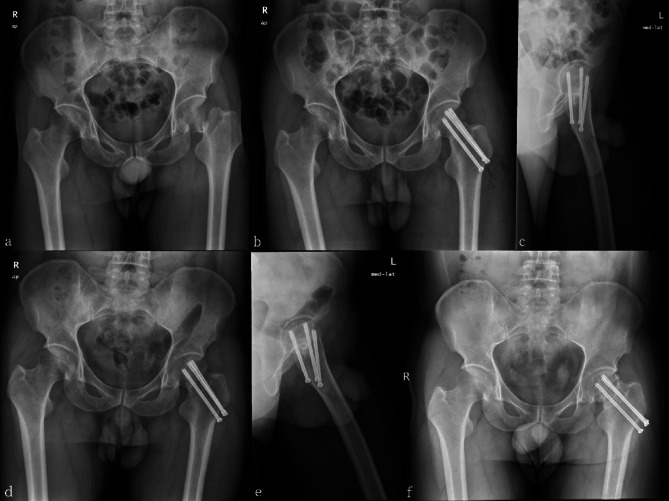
A 33-year-old male patient with left femoral neck fracture was treated with triple cannulated screws(TCS). (a) preoperative anteroposterior X-ray image. (b,c) postoperative radiographs anteroposterior and lateral images revealing satisfactory reduction of the fracture. (d,e) Anteroposterior and lateral X-ray images showing femoral neck shortening and screw withdrawal 1 month after operation. (f) Postoperative 16-month follow-up radiographs showing an increased degree of femoral neck shortening
